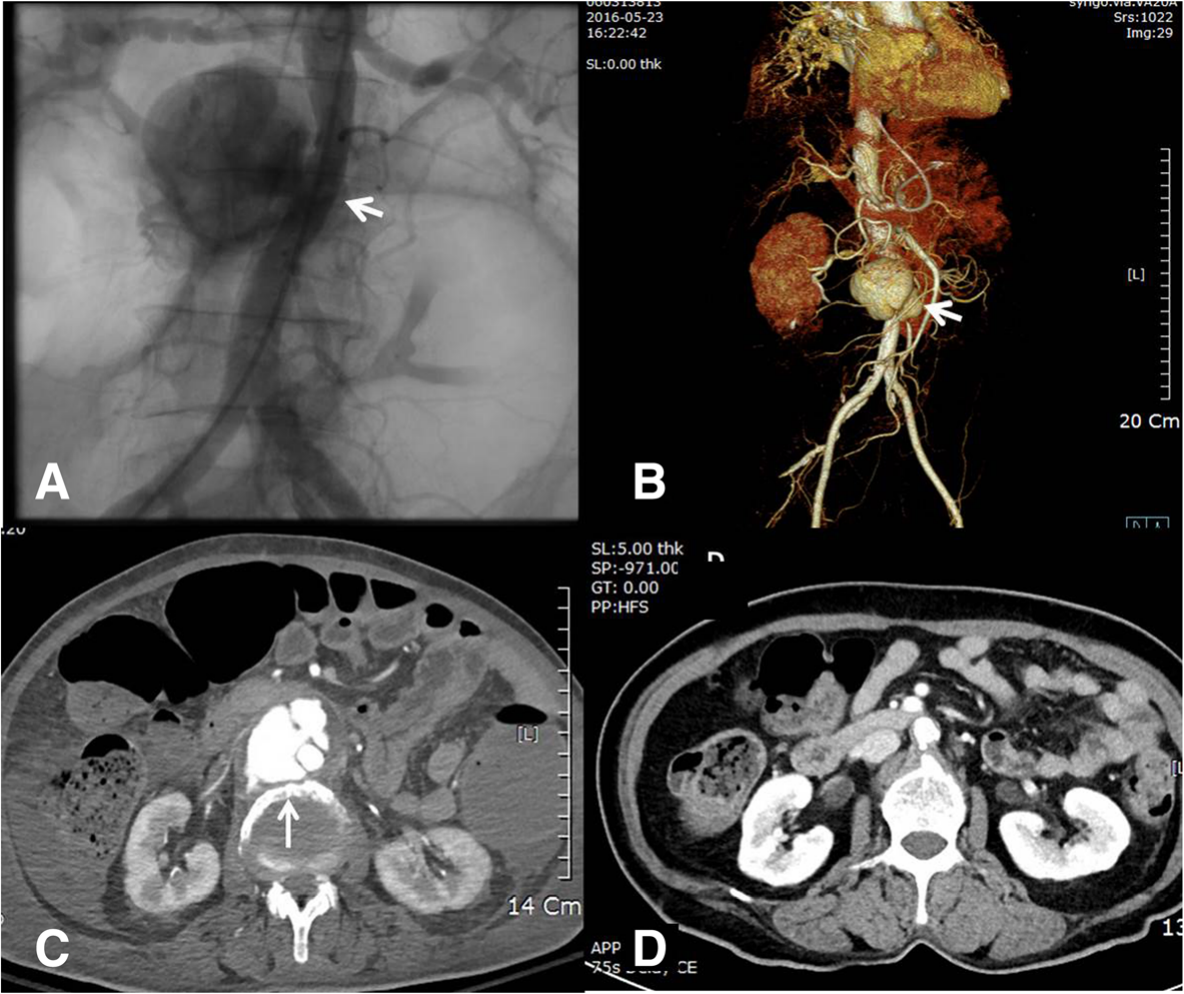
A large saccular aneurysm was detected in the infrarenal abdominal aorta by aortography (a, *white arrow*) and computed tomography (b and c, *white arrows*). The aneurysm had not been present 2 years previously (d)
